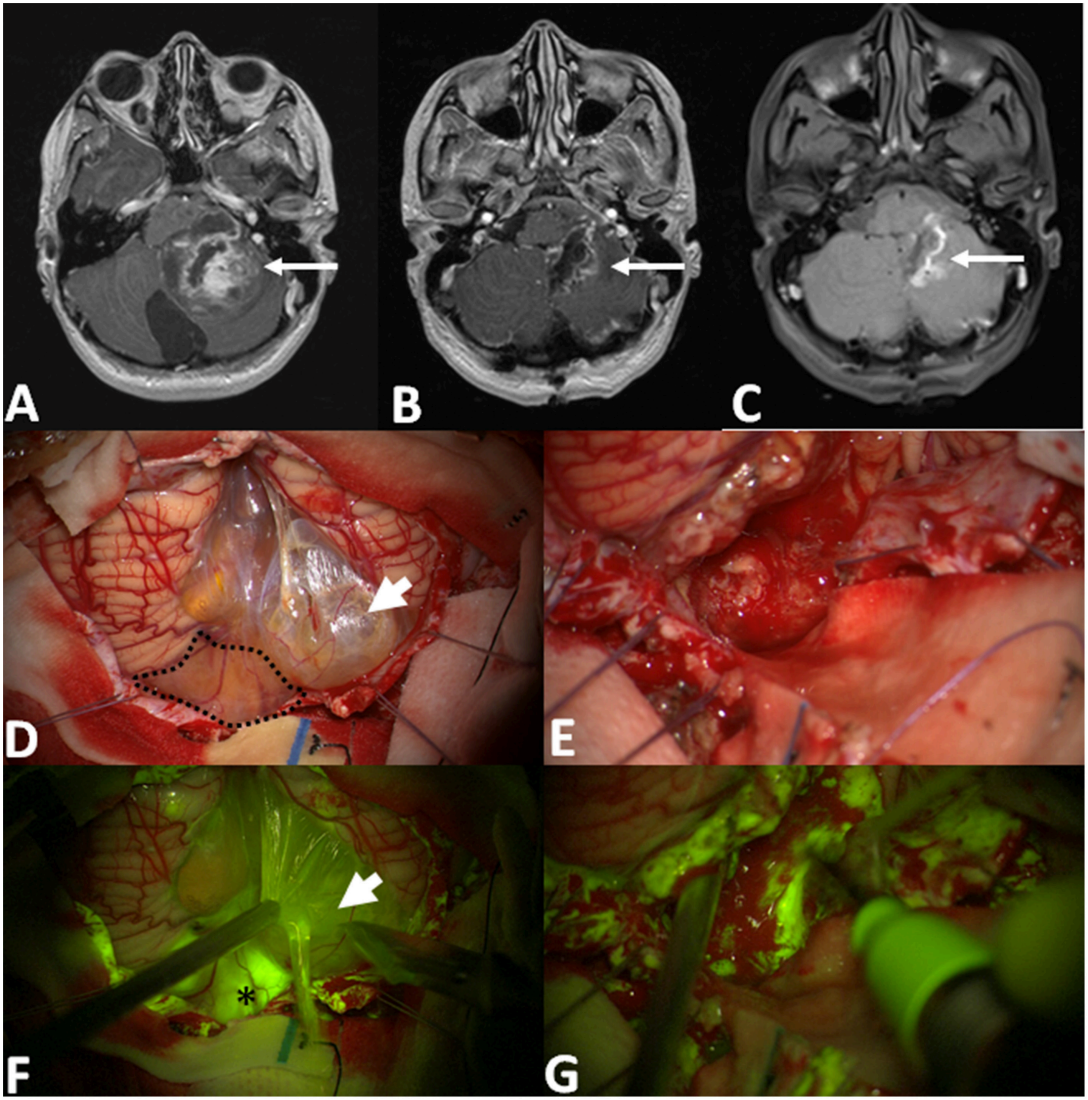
Pre-operative T1 with contrast axial scan (A) shows a huge posterior fossa pilocytic astrocytoma (arrow) with a gross-total resection, as detectable by post-operative post-Gadolinium T1 MRI in (B). The area of contrast-enhancement corresponds to the spontaneous hyperintense boundaries of surgical cavity (C). After dural opening (D,F), it is possible to appreciate a multilobulated cyst with a bright fluid (arrowhead in D, after its fenestration in F) and an inferior vermis nodular component (dotted line in D) with an intense fluorescein enhancement (asterisk in F). Intraoperative images, under white light (E) and Y560 filter (G), during tumor removal with cavitron ultrasound aspirator, showing a bleeding, friable tissue with inhomogeneous fluorescein enhancement.
